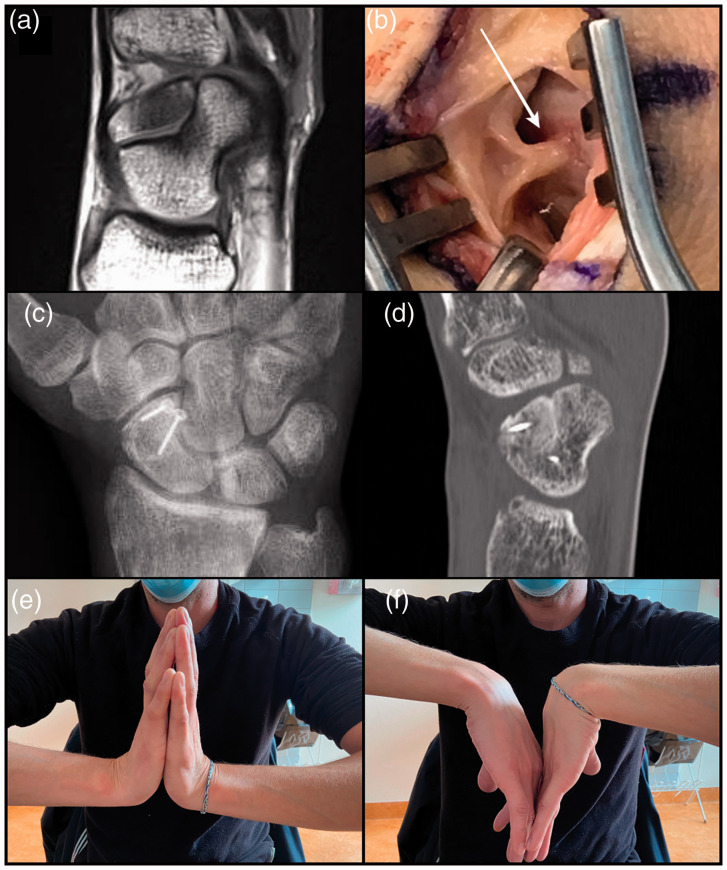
Patient 2. MRI showing the bipartite scaphoid, sagittal T1-weighted Turbo spin echo
